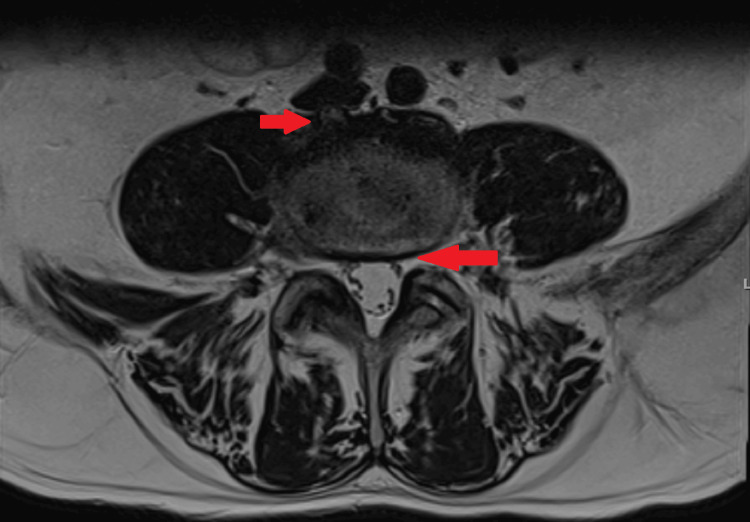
Follow-up (eight weeks) lumbosacral MRI (T2, transversal) showing resolution of the epidural collection
